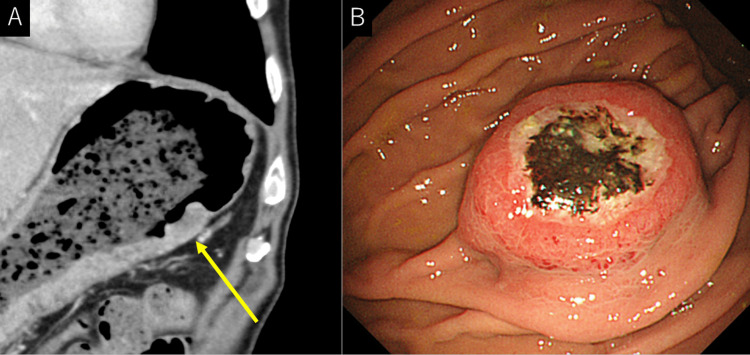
A representative case. A, contrast-enhanced CT, coronal image; B, endoscopy A case of a 65-year-old man suffering from lung cancer with multiple lung and lymph node metastases. CT showed an SMT lesion on the greater curvature of the gastric body (Figure 3A, arrow). Endoscopy revealed a self-destructing SMT at the top of the body of the stomach (Figure 3B). SMT, submucosal tumor
